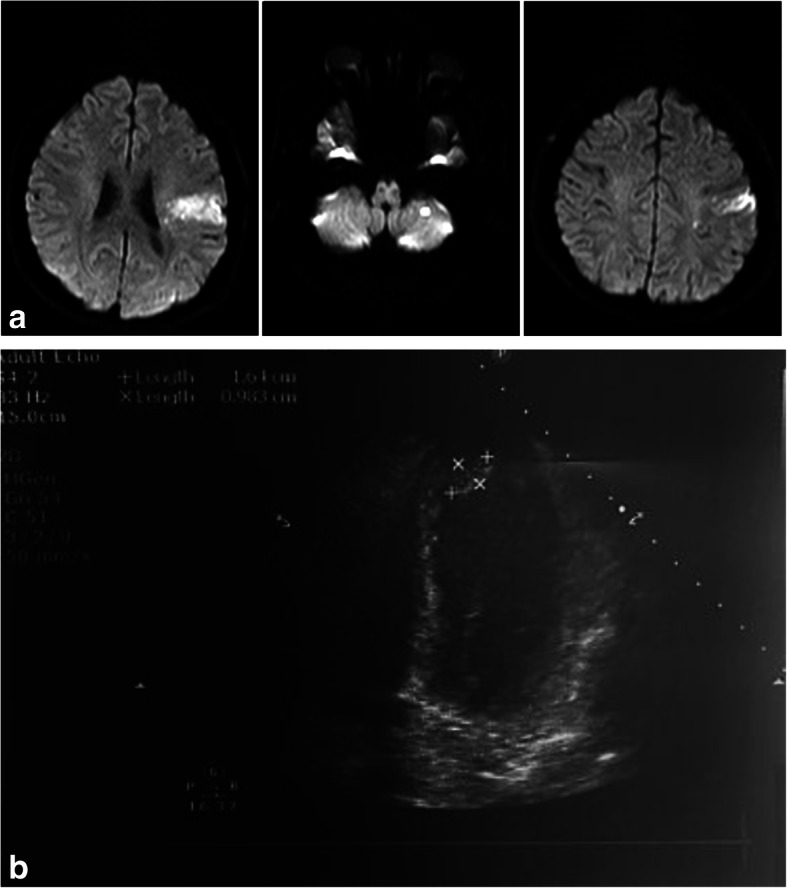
a MRI DWI of patient 10 with left insular and cerebellar infarction. b ECHO of patient 10 with fresh apical thrombus measuring 12 × 16 mm, EF 35%, akinetic all apical segment, mid-anterior wall, mid-anterior septum, and mid-posterior septum
